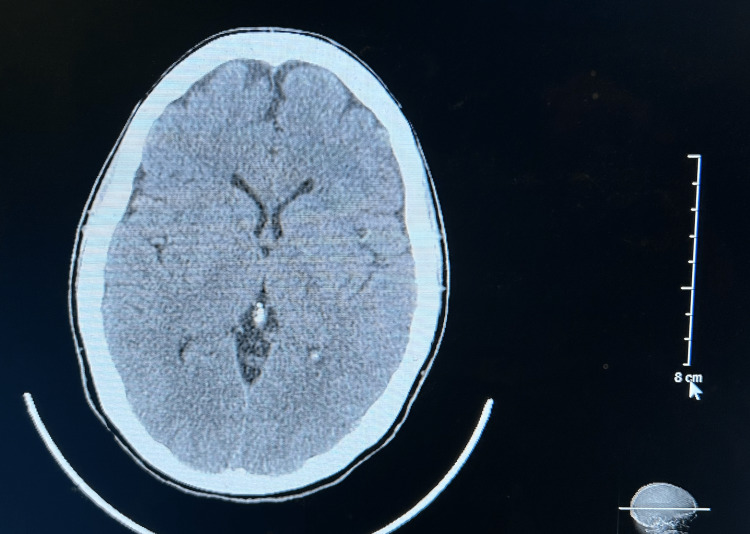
The CT scan of the brain (axial view) showed no relevant or significant changes
